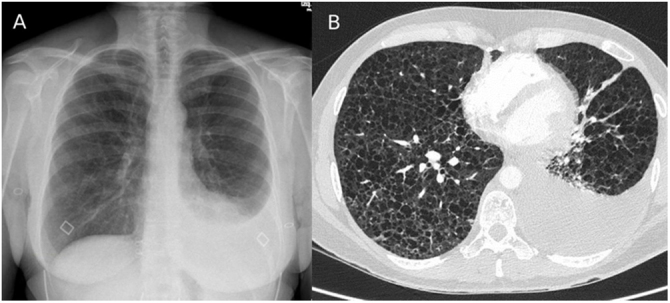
(A) Posteroanterior chest X-ray: Mild left pleural effusion. (B) AngioCT Chest: Countless pulmonary cysts distributed throughout the lung parenchyma with associated left pleural effusion.
